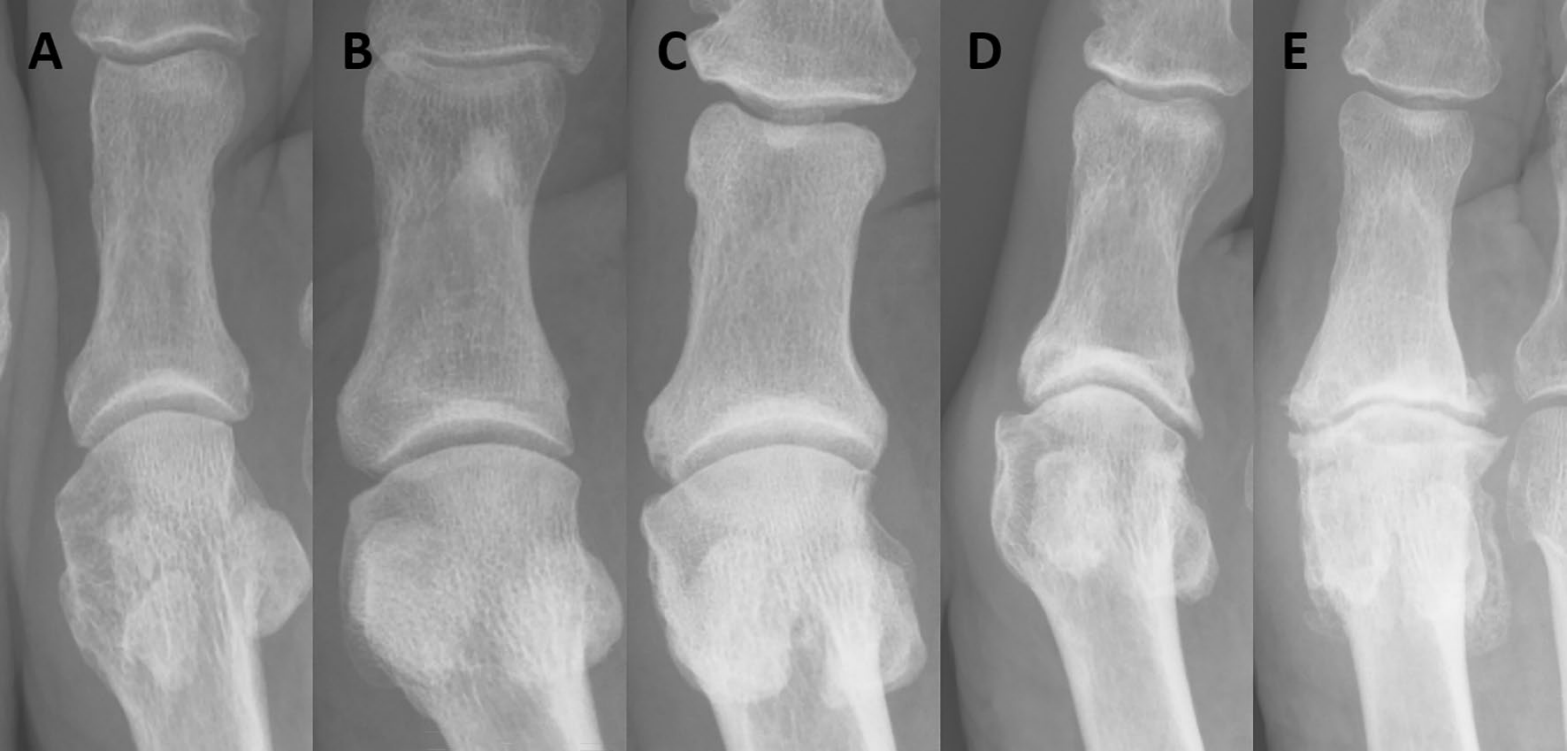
Radiographic OA of the MTP1 joint in patients with controlled acromegaly according to a modified Kellgren and Lawrence scoring method. Examples of the different scores of the modified Kellgren and Lawrence (KL) scoring system of the MTP1 joint in our acromegaly patients, based on the KL atlas of the hands, as described in several previous reports [49, 54, 55]. A KL score 0, B KL score 1, C KL score 2, D KL score 3, and E KL score 4. *MTP* metatarsophalangeal
